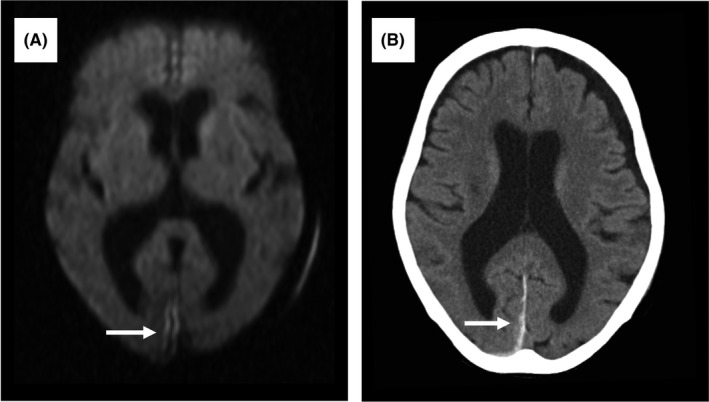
Imaging features of the right occipital region. A, Diffusion‐weighted magnetic resonance imaging on day 11 revealing an area of hyperintensity. B, Noncontrast computed tomography on day 19 revealing an area of hyperdensity
